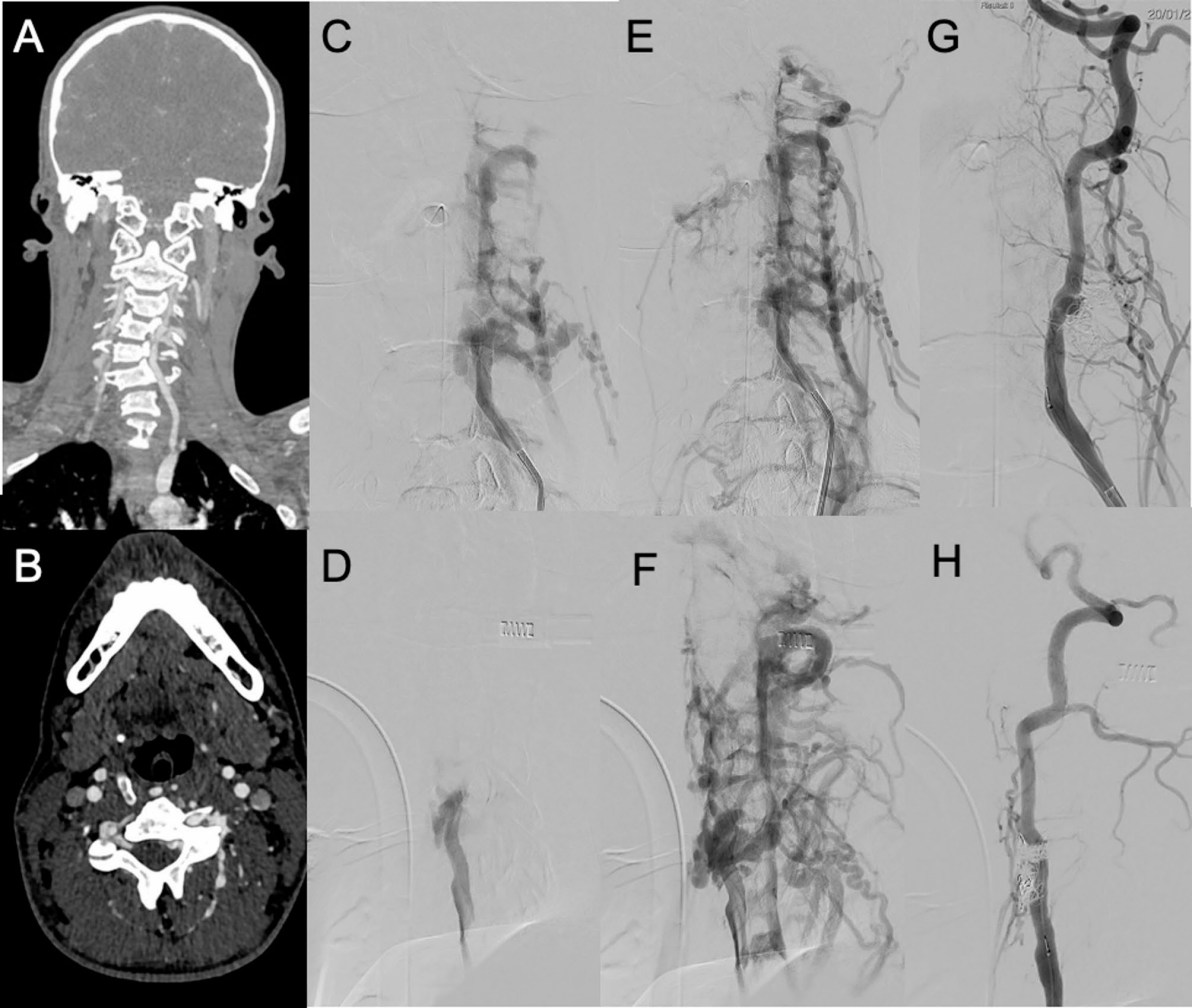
VAVF of an adolescent patient with neurofibromatosis 1 after a minor trauma. In A (coronal reconstructions) and B (axial native images) CTA shows asymmetric opacification of the cervical venous plexus, with early enhancement in the left side. In C, D, E and F the pre-operative DSA, performed while injecting the left vertebral artery, better demonstrates the presence of a VAVF between the left vertebral artery and the left cervical venous plexus, with evidence of dilated and tortuous arterialized veins (C, E: frontal views. D, F: lateral views). In G (frontal view) and H (lateral view) post-treatment results are shown, with complete occlusion of the fistula by coiling and preservation of the parent artery patency in the arterial phase
